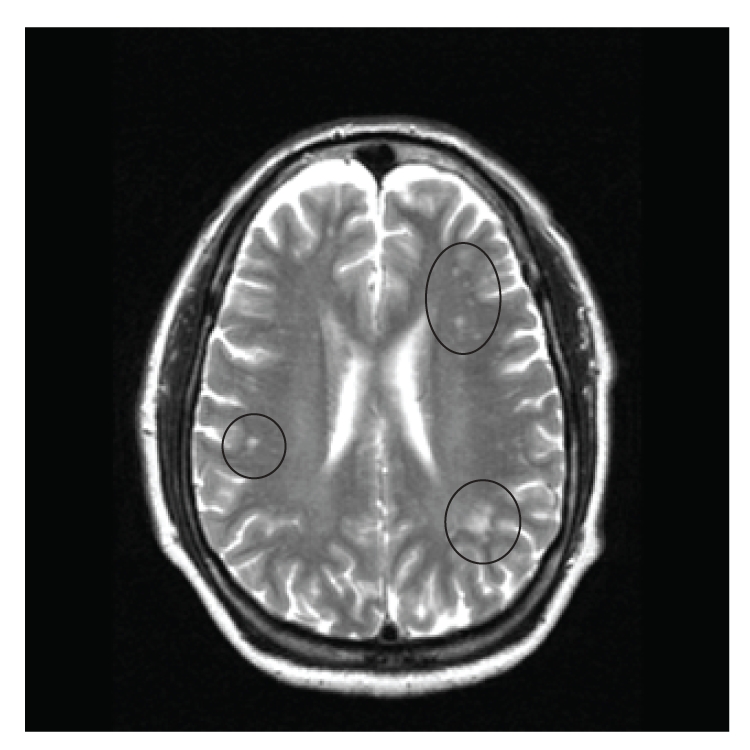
MRI image of the brain in an axial view showing the “pre-contrast T2 image”. Note the abnormal lesions (circled) in the per ventricular area suggesting white matter pathology.
